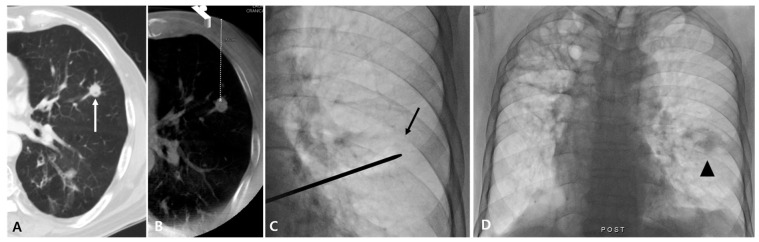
Case of fluoroscopy-guided PTNB with the aid of planning CBCT of a lung nodule in a 79-year-old man with lung metastatic adenocarcinoma that resulted in a complication. A diagnostic CT image (A) and planning CBCT image (B) show an 11 mm nodule (white arrow) in the left upper lobe with a long aerated lung length achieved by needle insertion (46 mm). A lesion depth was 75.6 mm (white dashed line). On fluoroscopy, the nodule is identifiable (black arrow) (C), and PTNB was performed twice. After PTNB, hemoptysis occurred, and a post-PTNB fluoroscopic image shows peritumoral consolidation, representing a hemorrhage (arrowhead) (D).
